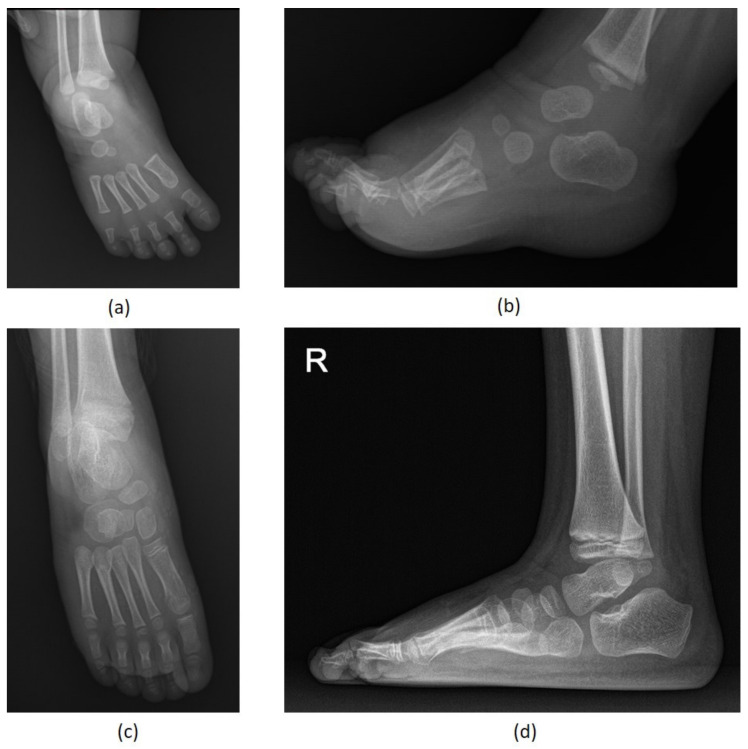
Preoperative anteroposterior (a) and lateral (b) radiographs of an early relapsed clubfoot with a talocalcaneal coalition that failed to repeat Ponseti casting; anteroposterior (c) and lateral (d) radiographs at the 38-month follow-up after the coalition resection and open reduction of the talonavicular joint.
